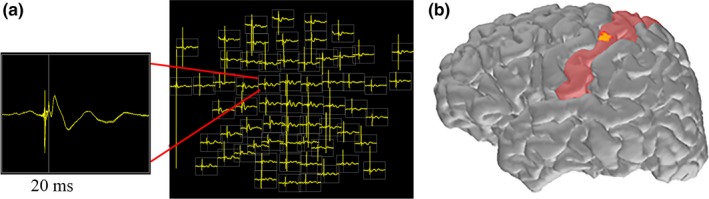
(a) Example EEG field map of averaged activity from ~100 right median nerve stimulations in a single subject. The N20 peak is confirmed through visual analysis. (b) Electrical source localization is performed utilizing the patients MRI data and co‐registered electrode coordinates. Visual inspection of the N20 peak (yellow) confirms localization to the postcentral gyrus (primary sensory cortex) hand representation area
